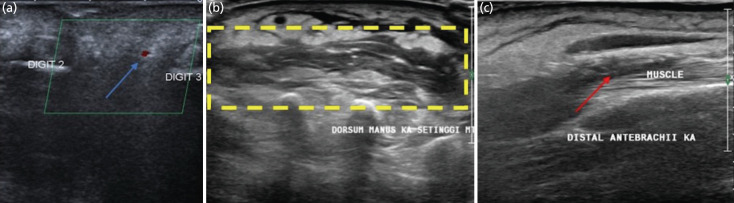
(a) Doppler ultrasonography (USG) on dorsal hand showed no blood flow in radial nor ulnar side of proper digital artery of second finger, while its visible in third finger (blue arrow). (b) Focal multiple anechoic / hypoechoic area in dorsal of the hand on metacarpals level (yellow dashed square) and (c) hypoechoic in intermuscular area in distal forearm (red arrow).
